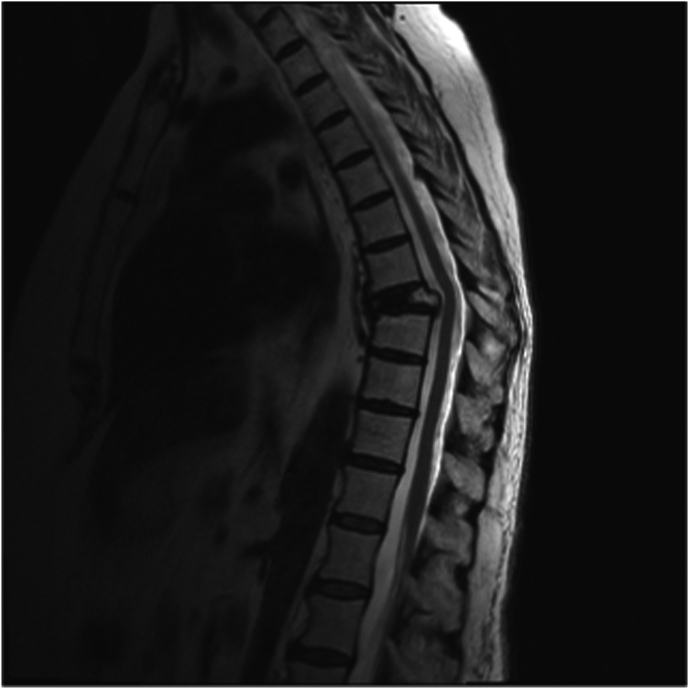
Sagittal non-fat saturated T2 weighted image MRI of the thoracic spine 5 months prior to intervention showing normal thoracic spinal cord without abnormal signal. No evidence of cord edema.
